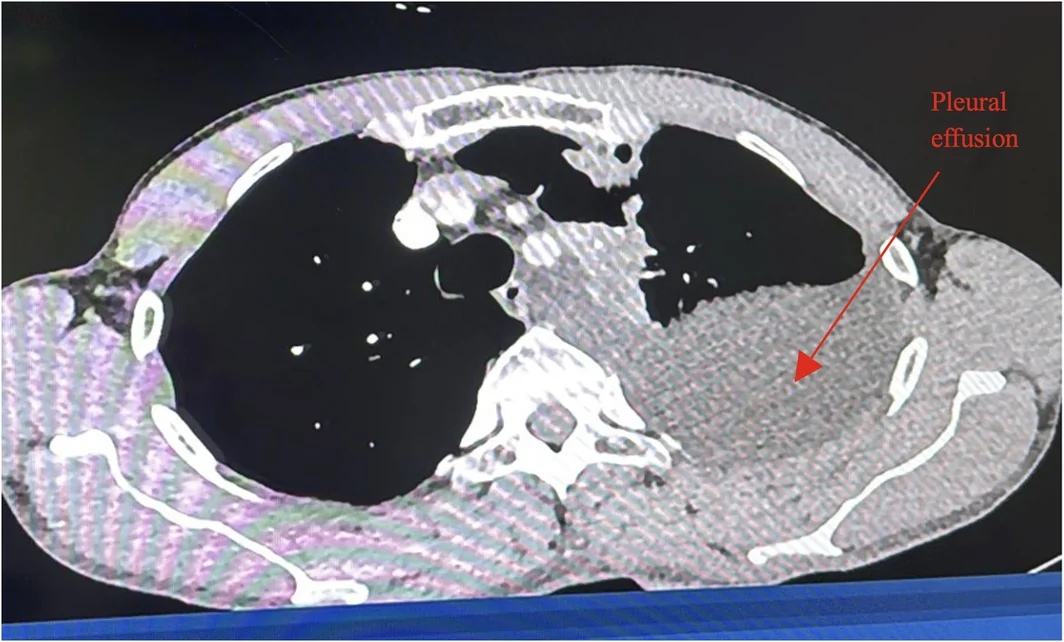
CT image following EUS‐FNA demonstrating a moderate to large left‐sided pleural effusion (secondary to chylothorax).
